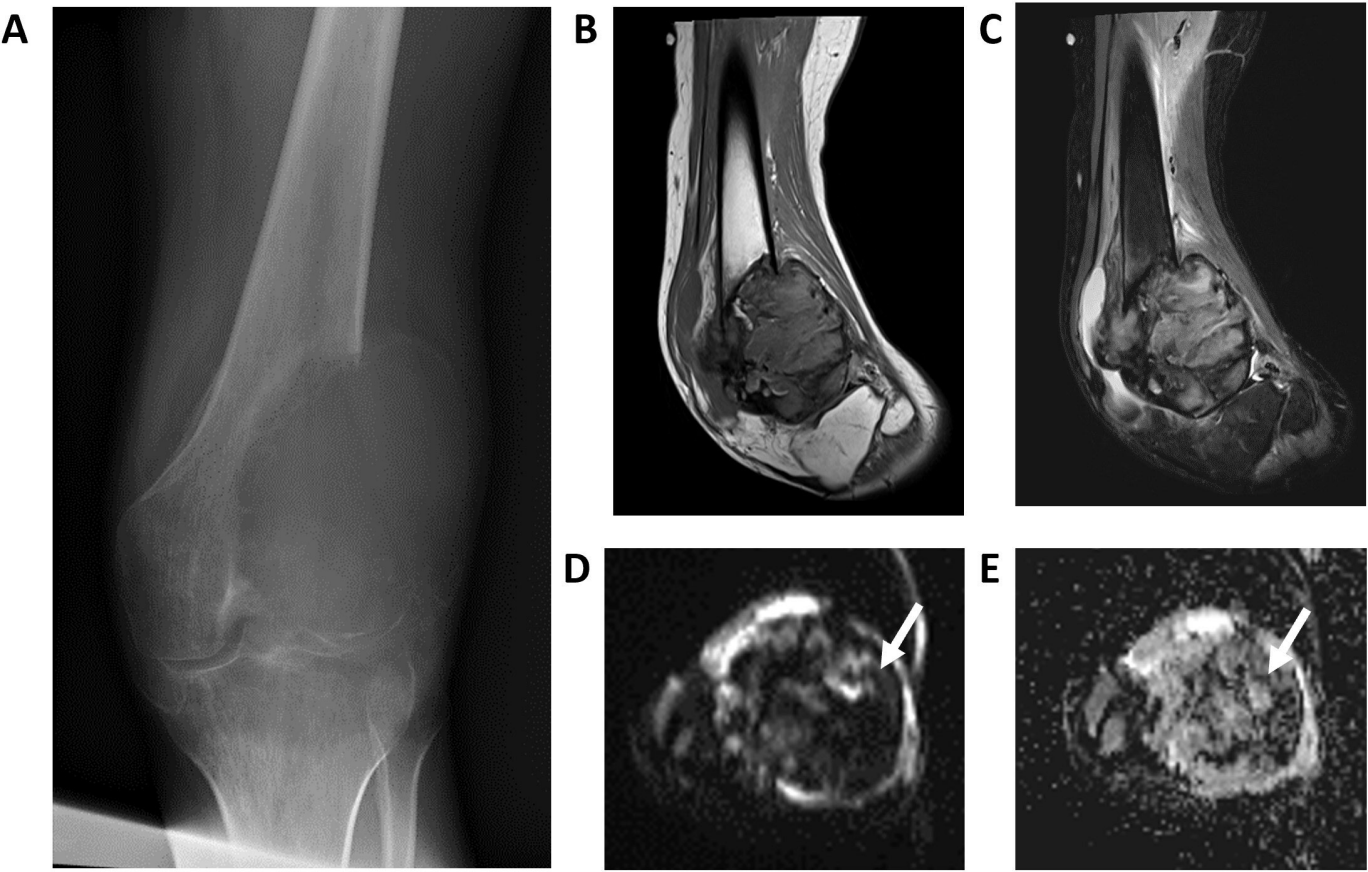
Giant cell tumor on anteroposterior X-ray (A), sagittal *T*
